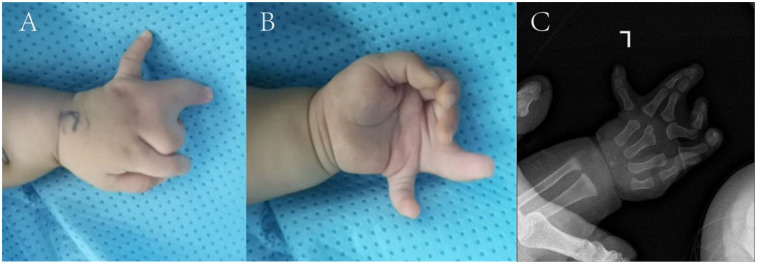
(A,B) Preoperative appearance and, (C) x-ray films.
